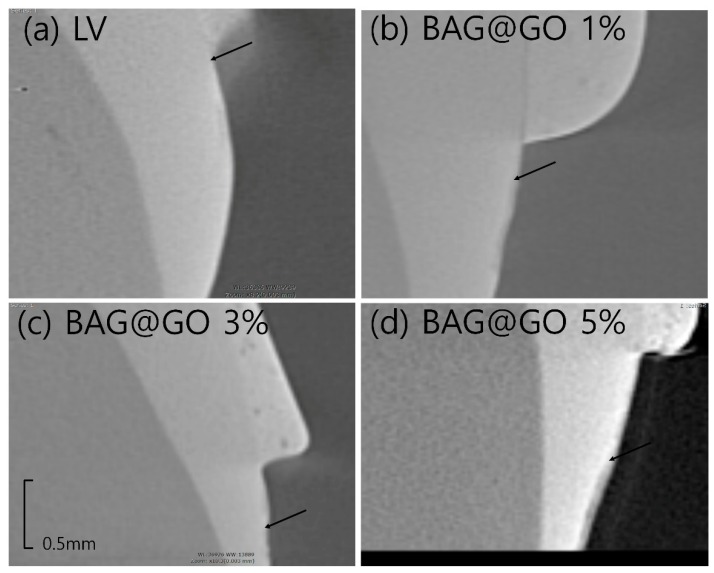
Anti-demineralization point (black arrow) of the LV and BAG@GO orthodontic bonding adhesive via CBCT. (a) LV; (b) 1 wt.% BAG@GO; (c) 3 wt.% BAG@GO; and (d) 5 wt.% BAG@GO.
